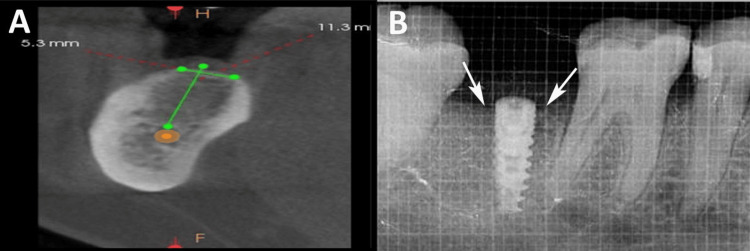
Measurement of alveolar bone levels in millimeters at preoperative phase (A) and assessment of crestal bone levels using grid method at three months on intraoral periapical radiographs (white arrows) (B). Original images of a patient from the study, used with patient's consent. Measurement unit of grid: 1 grid = 1 mm.
